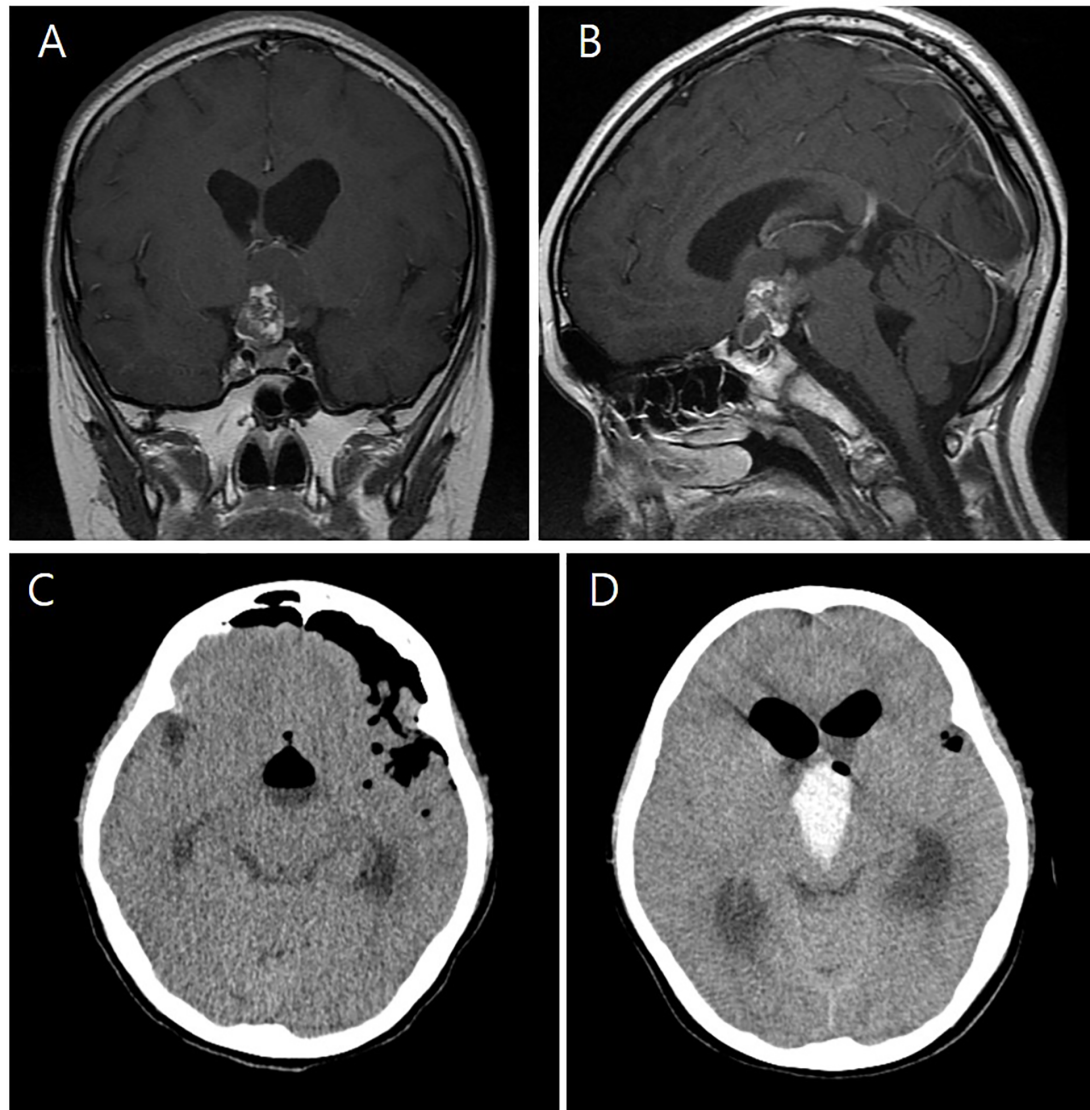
Preoperative T-1 enhanced coronal (A) and sagittal (B) images of an eight-year-old girl showing a 3.4 cm × 2.5 cm × 1.7 cm craniopharyngioma with cystic and solid components and calcification in the suprasellar area. Immediate postoperative brain CT shows no abnormal findings (C). However, on the first postoperative day, intraventricular hemorrhage was found in the third ventricle (D).
